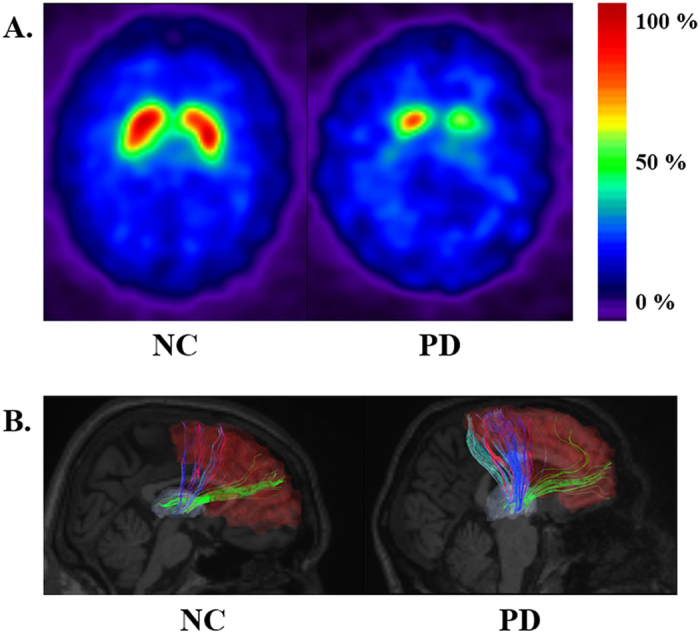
Representative SPECT images and fiber tracts obtained from DTI of NC and PD cases. (A) The SPECT images were representative slices taken from registered 3D volumes. Note the difference in putamen and caudate regions. (B) Fiber tracts obtained from DTI tractography focusing on associate cortex – thalamus connection. Associative cortex was colored in red and thalamus was colored in light purple. Note the increased number of fiber connecting the regions in the PD case compared to NC case.
